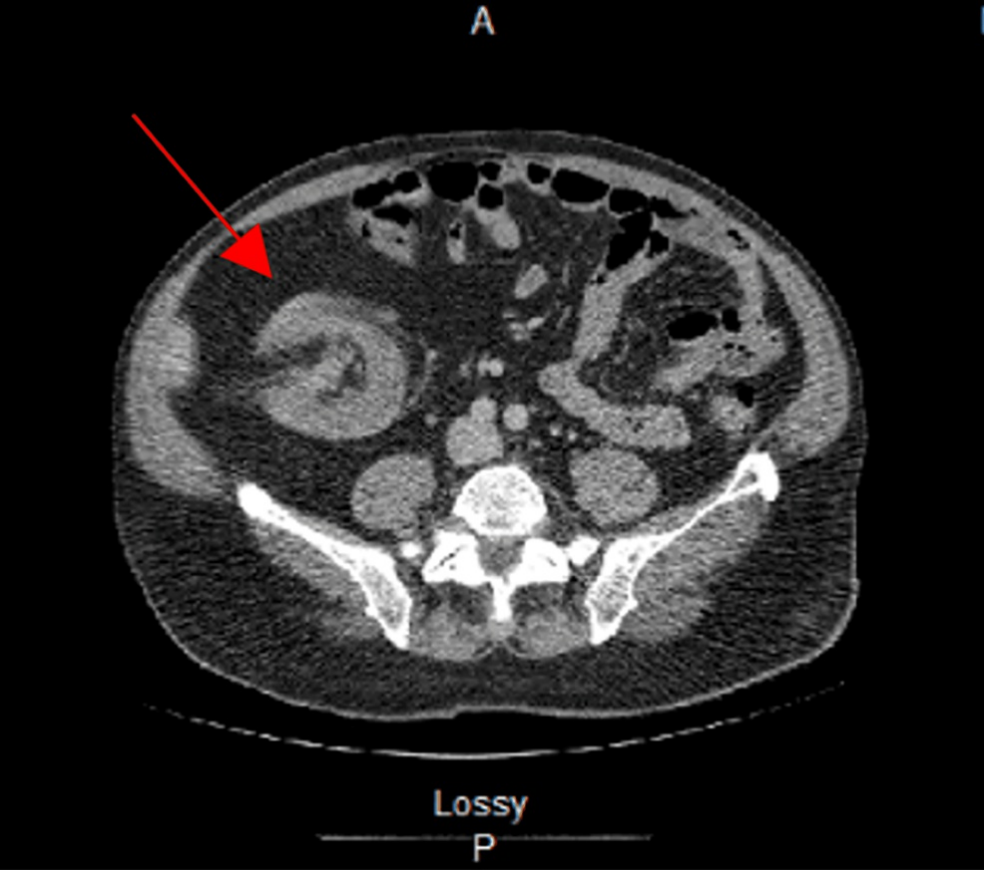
CT imaging with IV contrast demonstrated a proximal transverse colon obstruction resulting in a "bowel within a bowel" configuration which is consistent with intussusception.
